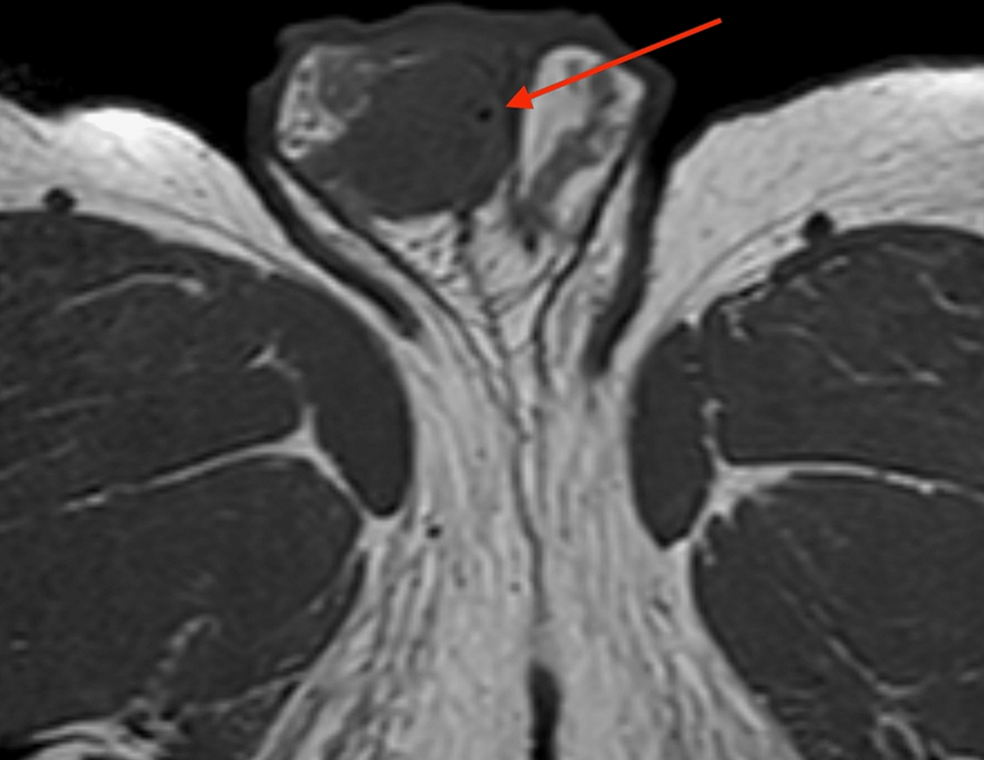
T1-weighted transversal MRI of the pelvis Image of the scrotum and right testicle and transversal view of both thighs showing a punctiform signal gap in the tumor center in the right testicle (marked with the arrow)
